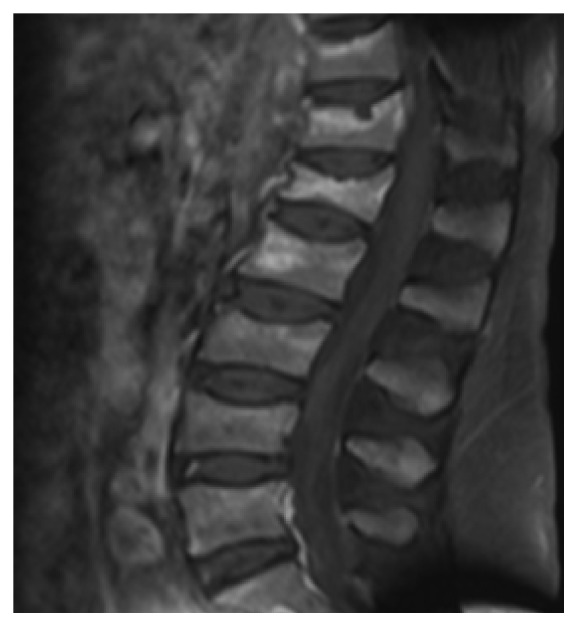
Lumbar spine metastasis on MRI.
